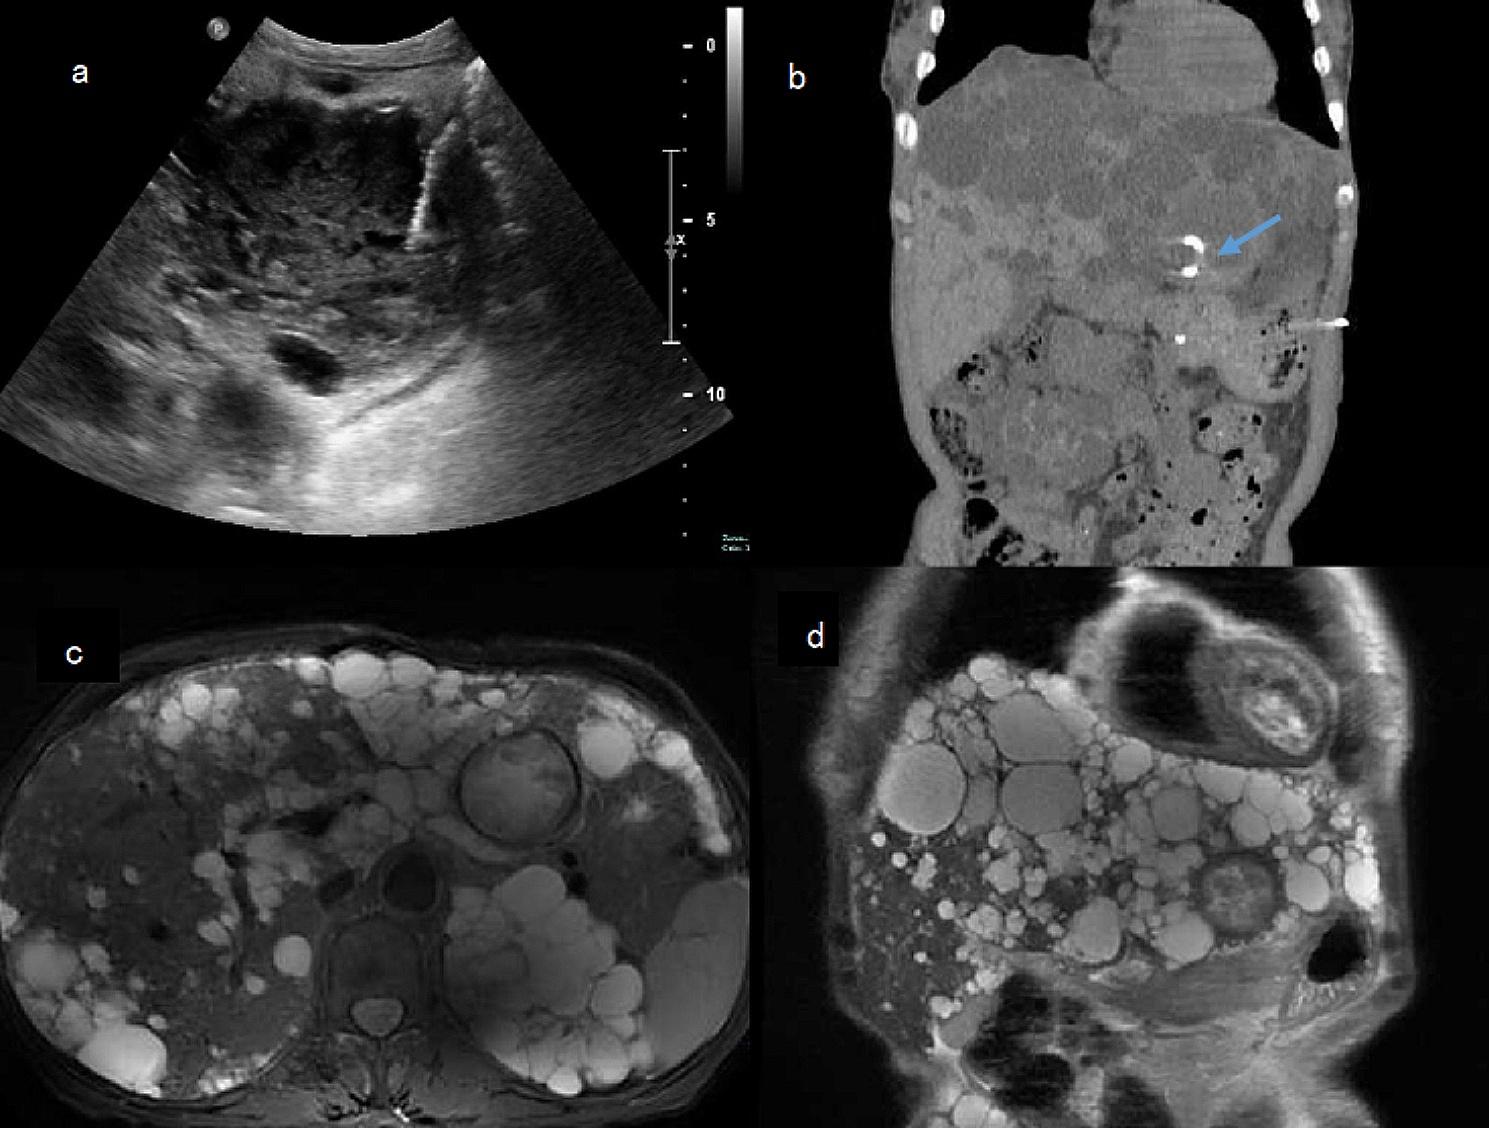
Puncture and insertion of the drainage tube. a: Ultrasound-guided puncture of the infected liver cyst was performed, and an 8-Fr pigtail drainage tube was successfully inserted. b: The drainage tube shadow (arrow) is seen in the cystic lesion of the left lobe of the liver, and the lesion was smaller than before. c: Puncture MRI examination of the T2 cross-section after drainage. d: MRI examination of the T2 sagittal plane after puncture drainage
